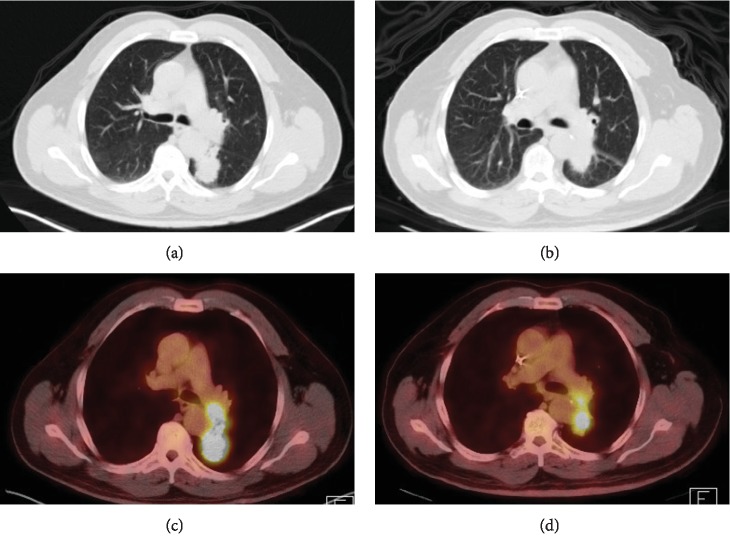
CT and fused PET/CT imaging (axial). (a, b) Staging CT and fused PET/CT of the base of the skull to midthigh showing a FDG avid mass in the left lower lobe of the lung with adjacent left hilar lymphadenopathy. Max SUV 14.83. (c, d) Restaging CT and fused PET/CT of the full body approx. 4 months after initiation of chemotherapy showing reduction in size of FDG avid mass in the left lower lobe of the lung with adjacent left hilar lymphadenopathy.
